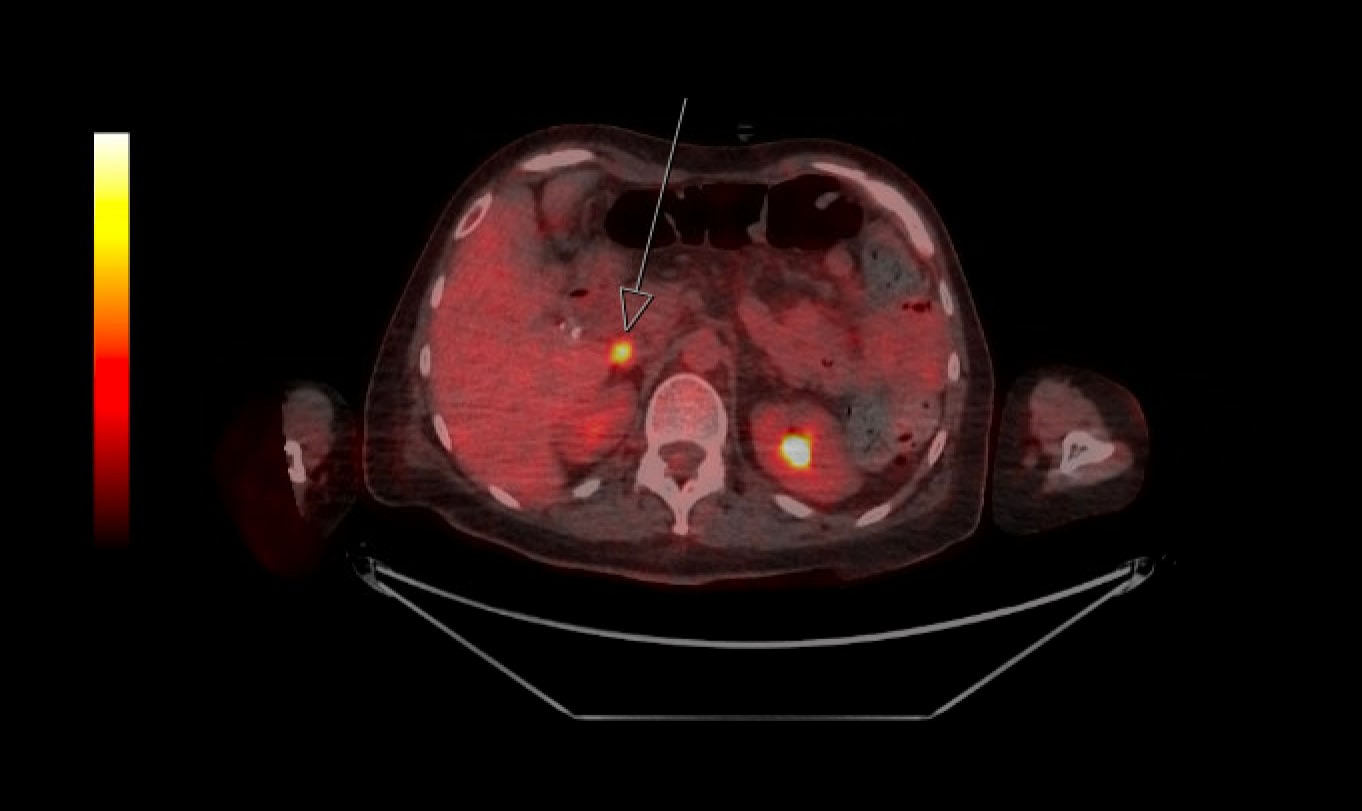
Positron emission tomography image showing isolated hypermetabolic portocaval node (depicted by arrow; SUVmax 10.0).
